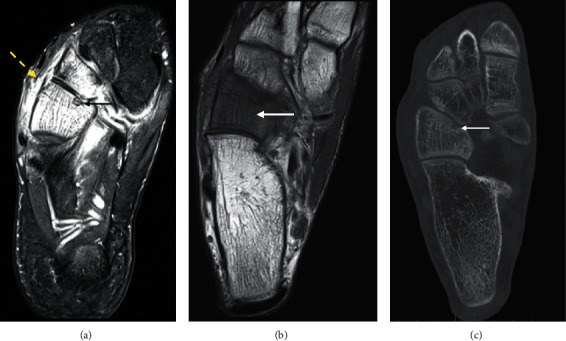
Cuboid osteoid osteoma in a 17-year-old presenting with a 2-month history of foot pain. Axial PDFS (a) of the foot shows diffuse marrow oedema-signal in the cuboid and lateral cuneiform with adjacent synovitis and soft tissue oedema. There is T1 marrow replacement of the cuboid ((b), arrow). A fluid-hyperintense nidus, best seen on the fluid-sensitive sequence, is noted in the medial border of the cuboid, with extension into the articular surface (arrow), accounting for the effusion and reactive synovitis (A yellow dash). The nidus is confirmed on the CT ((c), arrow), demonstrating central calcification, and distinguishes the diagnosis of osteoid osteoma from infection.
